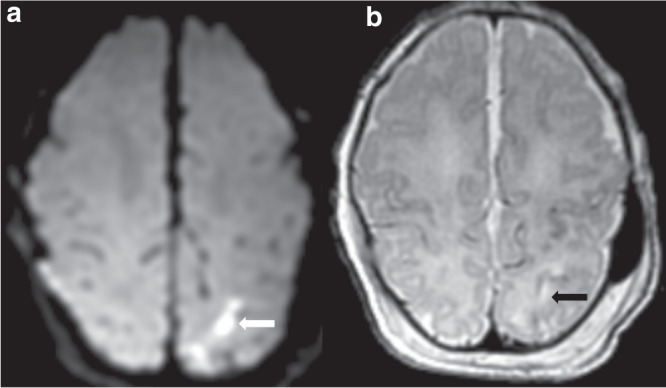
Acute arterial watershed pattern of hypoxic ischemic brain injury. a DWI demonstrating restricted diffusion (white arrow) and b T2 demonstrating hyperintensity (black arrow) in the left superior parietal white matter.
